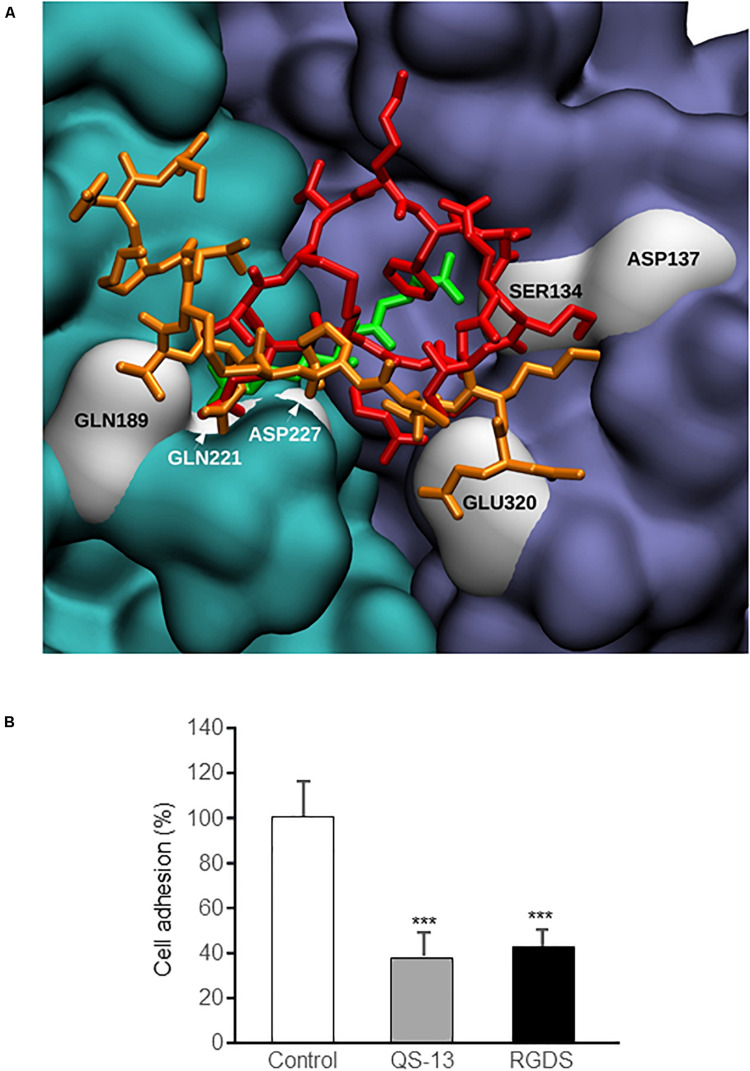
Comparison of QS-13 and RGD theoretical binding sites on α_5_β_1_ integrin and *in vitro* confirmation. (A) α_5_β_1_ integrin is represented using the Quick Surface scheme and a color code related to the nature of the subunit (cyan for α_5_ and magenta for β_1_). The best docking poses of the two QS-13 conformations are superimposed with the conformation of the RDG peptide (from the experimental complex corresponding to the PDB ID 3VI4 solved with X-ray experiments) and depicted with a color coded (first QS-13 conformation extracted from the MD in red, the second in orange and RGD peptide in green) Licorice representation. Residues from the integrin making contacts with QS-13 (as evidenced in Figure 3) are highlighted with white surface representation and labeled. (B) HUVECs were pre-incubated for 30 min with culture medium alone (control), culture medium supplemented with RGDS peptide (20 μg/mL) or with QS-13 (20 μg/mL) and adhesion was measured. Three independent experiments were carried out. The histogram represents the means ± SD of the more representative one. *n* = 8, p* < 0.001.
